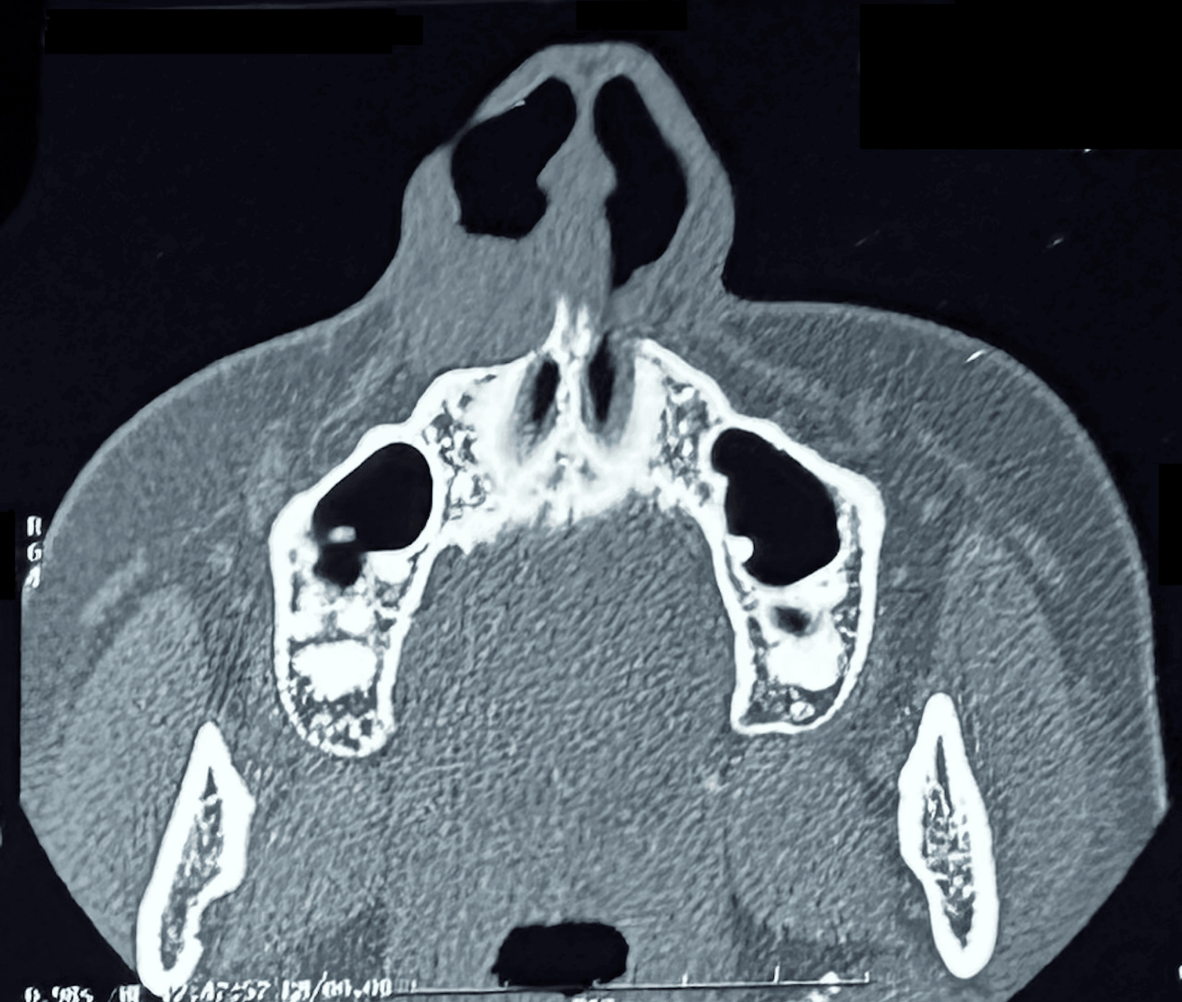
CT scan of paranasal sinuses (axial section) showing rounded mild hyper dense cystic lesion measuring ~ 2 x 1.1 cm in right nasolabial soft tissue plane. There is mild compression remodelling of underlying maxillary bone with no significant erosions
